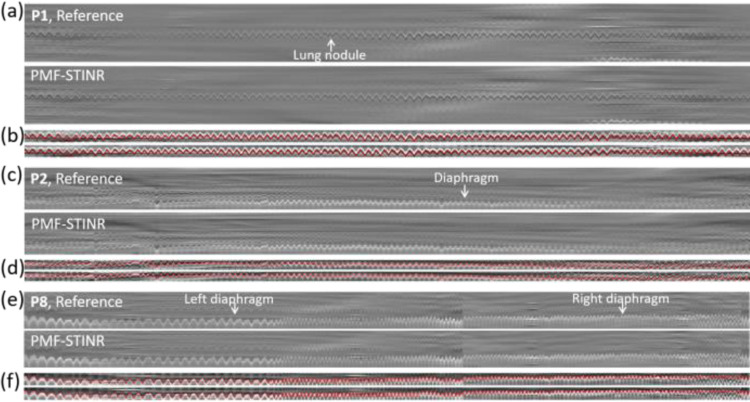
Comparison between the reference and the solved motion trajectories, respectively extracted from cone-beam projections and the DRRs via the Amsterdam Shroud (AS) method. (a–d) P1 and P2 were scanned by the full-fan mode where the tracking objects (P1: lung nodule, P2: diaphragm) were visible in all projections. (e–f) P8 was scanned by the half-fan mode where the tracked objects (diaphragms) were only visible in parts of the projections. Thus, the left and right diaphragms of P8 were tracked consecutively based on their visibility. Figs. 10-a&c&e show the AS images derived from the projections and DRRs. Figs. 10-b&d&f show the vertically cropped, z-score normalized regions containing the tracked objects, on which the motion trajectories (red lines) were extracted for quantitative evaluation.
